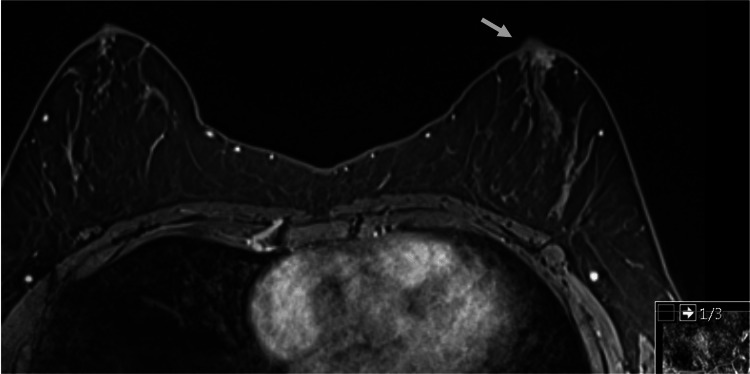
Breast MRI showing a nodular area in the retroareolar region of the left breast, measuring 10 mm.
